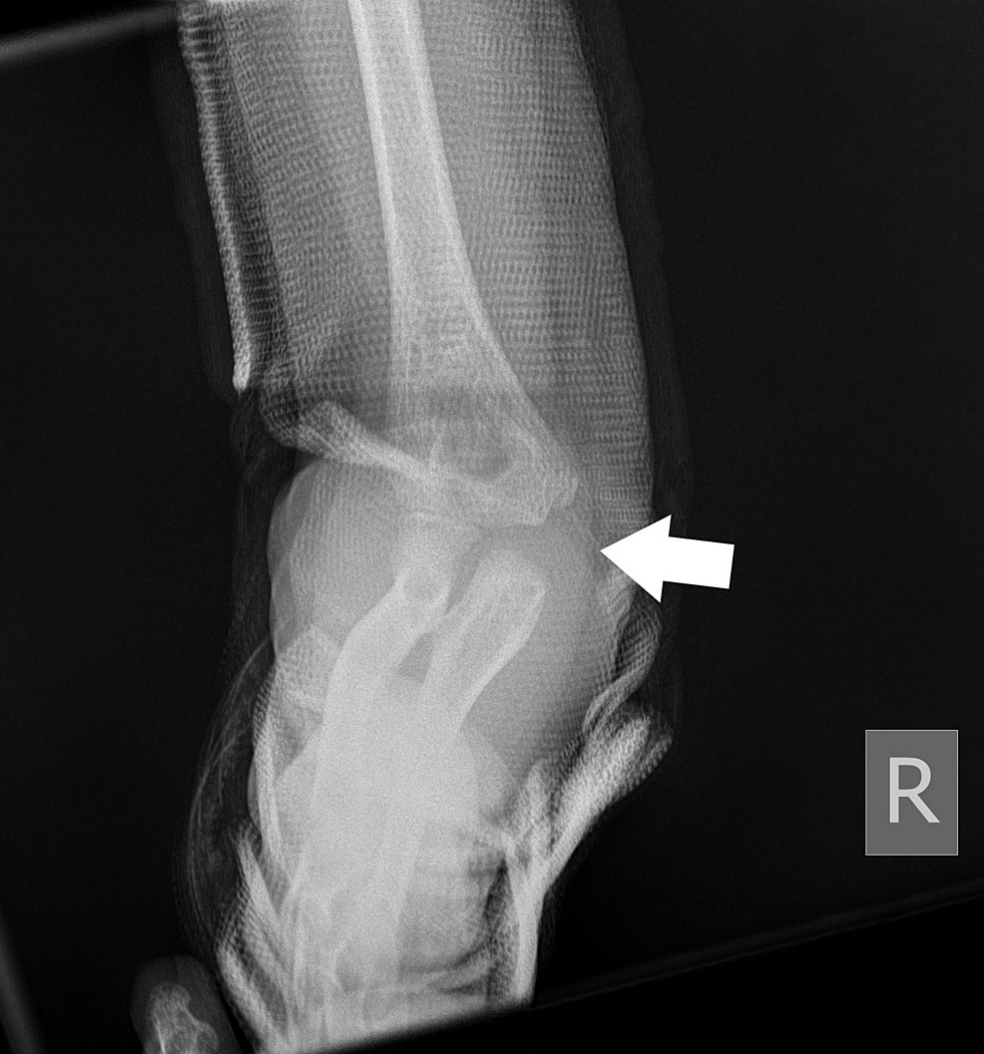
Postoperative x-ray of right (R) elbow after closed reduction (white arrow) (view 2).
